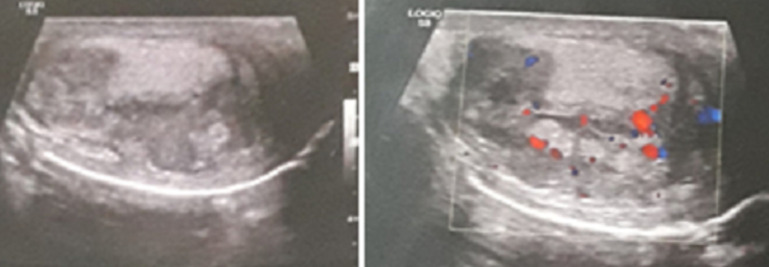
scrotal ultrasonography shows hypoechoic mass lesion in the right testis
